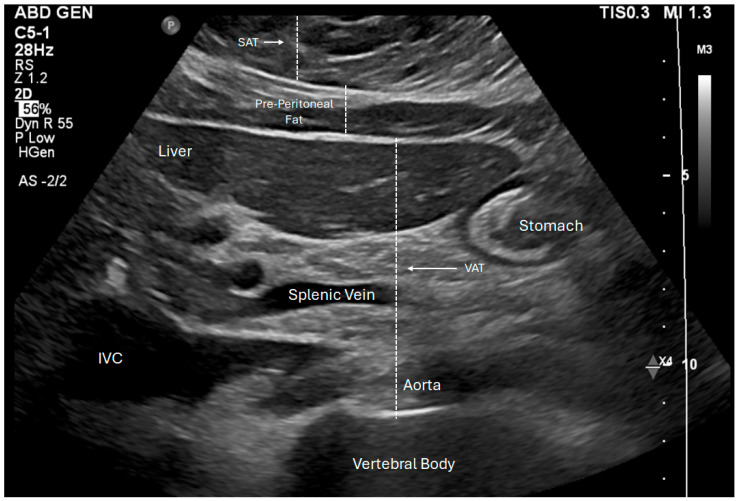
A transabdominal ultrasound image demonstrating regional landmarks: A transabdominal gray-scale ultrasound image of the midline upper abdomen acquired with a low-frequency (high-depth) ultrasound probe in a transverse position. Typical anatomic structures that are seen in the field of view when measuring VAT are annotated, including the liver, stomach, inferior vena cava (IVC), splenic vein, aorta, and vertebral body. In this image, visceral adipose tissue (VAT) is measured from the peritoneum (thin, echogenic, linear structure just superficial to the liver, to the posterior aspect of the aorta), as opposed to the method described in Figure 1. The different methods of VAT measurement highlight the potential pitfalls of comparing reference values or subcutaneous adipose tissue (SAT)/VAT ratios between different studies. Figure courtesy of Jordan Perchik MD.
